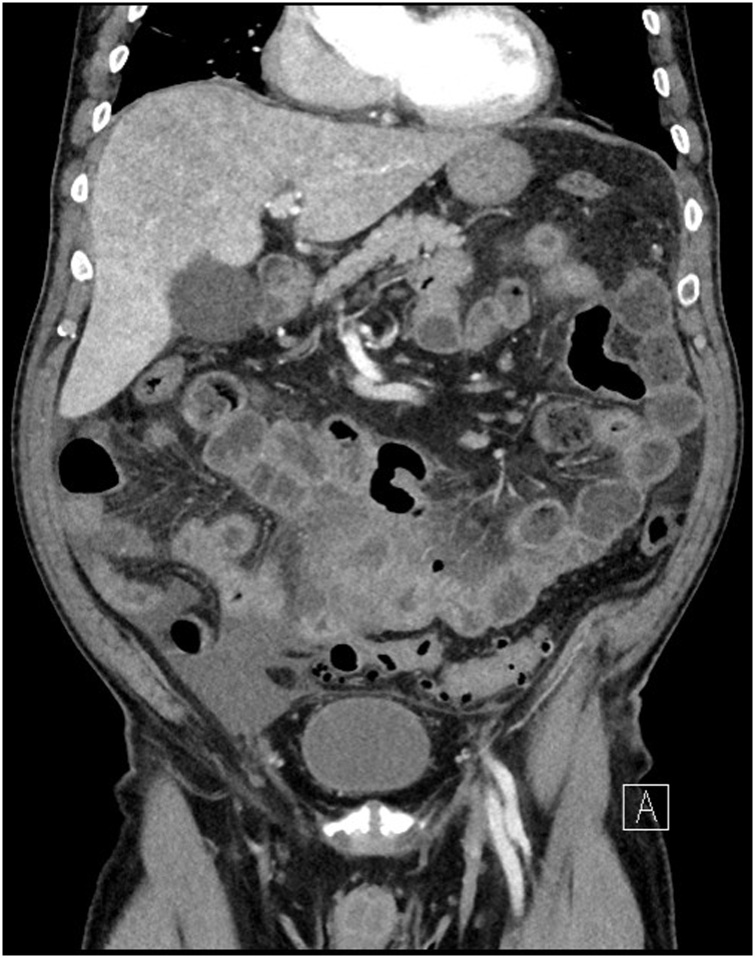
Coronal image from a contrast enhanced CT scan showing extensive bowel wall thickening and mesenteric fat stranding with free liquid in the right pelvic area.
